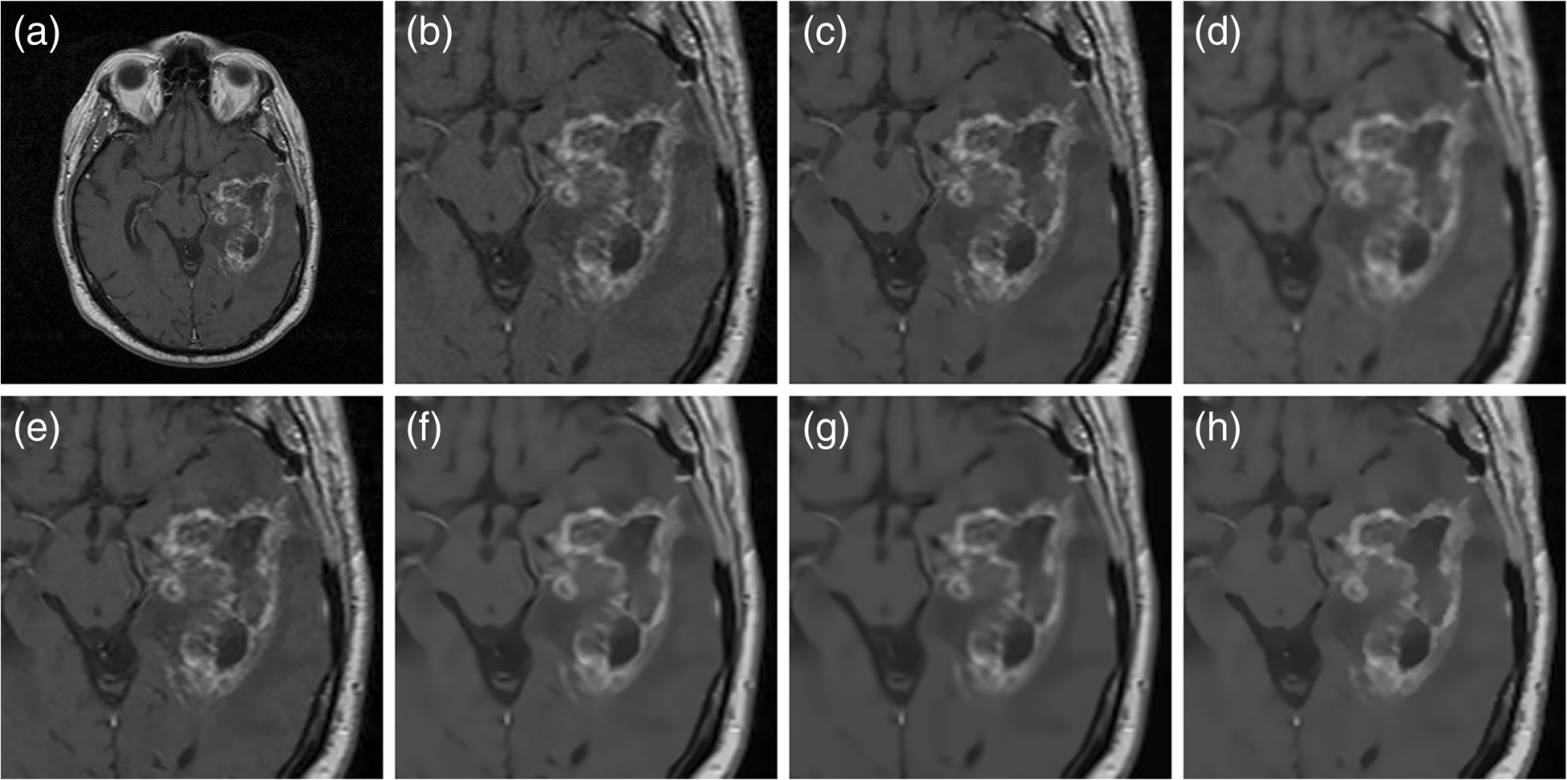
Comparison of the restoration of clinical T1-weighted MR images with brain tumors. a Input MR image. b Input image in magnified view. c IBF. d ADF. e LMMSE. f NLM. g BM3D. h Proposed
